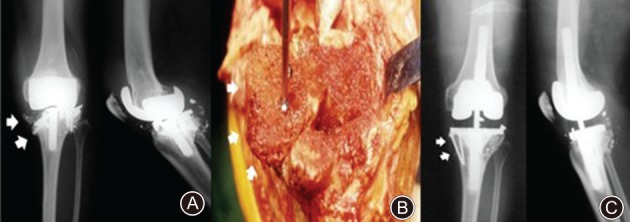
(A) Anteroposterior and lateral radiographs show a severe osteolysis in proximal tibia and distal femur with metal breakage (white arrow). (B) The femoral head allograft was stabilized with screws at the proximal tibia (white arrow). (C) The allograft remained intact with minimal resorption at 6 years after surgery (white arrow).
